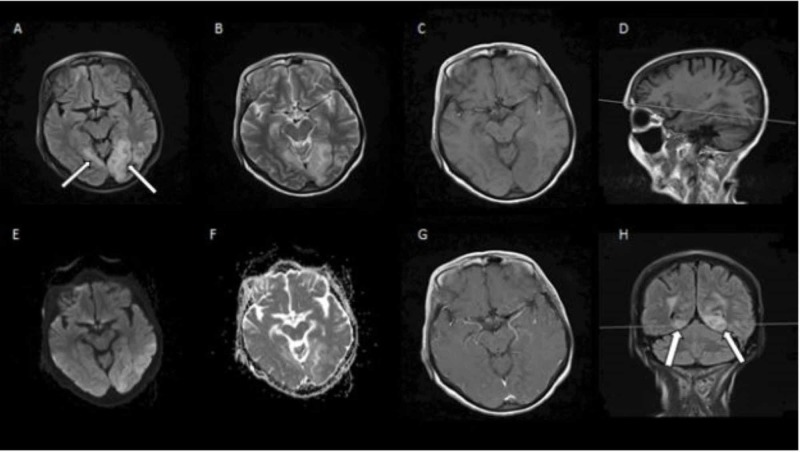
MRI of the brain with and without intravenous contrast A: axial T2 FLAIR; B: axial T2; C: axial T1 FLAIR; D: sagittal T1 FLAIR; E: axial DWI; F: axial T2 ADC; G: axial T1 with contrast; H: coronal T2 FLAIR Cortical and subcortical T2 FLAIR hyperintensity involving the bilateral posterior temporal lobes and the left occipital lobe (arrows), representing posterior reversible encephalopathy syndrome. There is no evidence of acute infarction. No enhancing lesions are identified MRI: magnetic resonance imaging; FLAIR: fluid-attenuated-inversion-recovery; DWI: diffusion-weighted imaging; ADC: apparent diffusion coefficient
